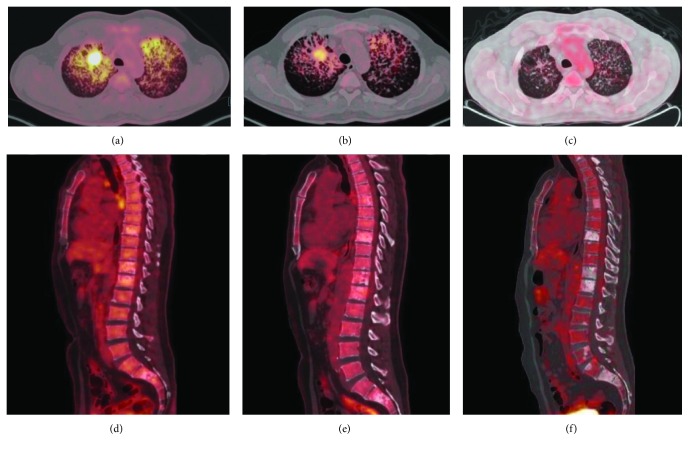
18-FDG PET CT fusion images: axial and sagittal slices. (a, d) Right upper lobe mass with abnormally increased metabolism with surrounding lymphangitic carcinomatosis on both upper lobes with increased metabolic activity as well. On sagittal slices, multiple mixed type (blastic/lytic) metastatic lesions affecting axial skeleton. (b, e) Images obtained 7 months later showed marked decrease on metabolic activity on both pulmonary and skeletal lesions. (c, f) Images obtained 11 months from first study demonstrate the absence of metabolic activity on both primary site and spinal lesion.
